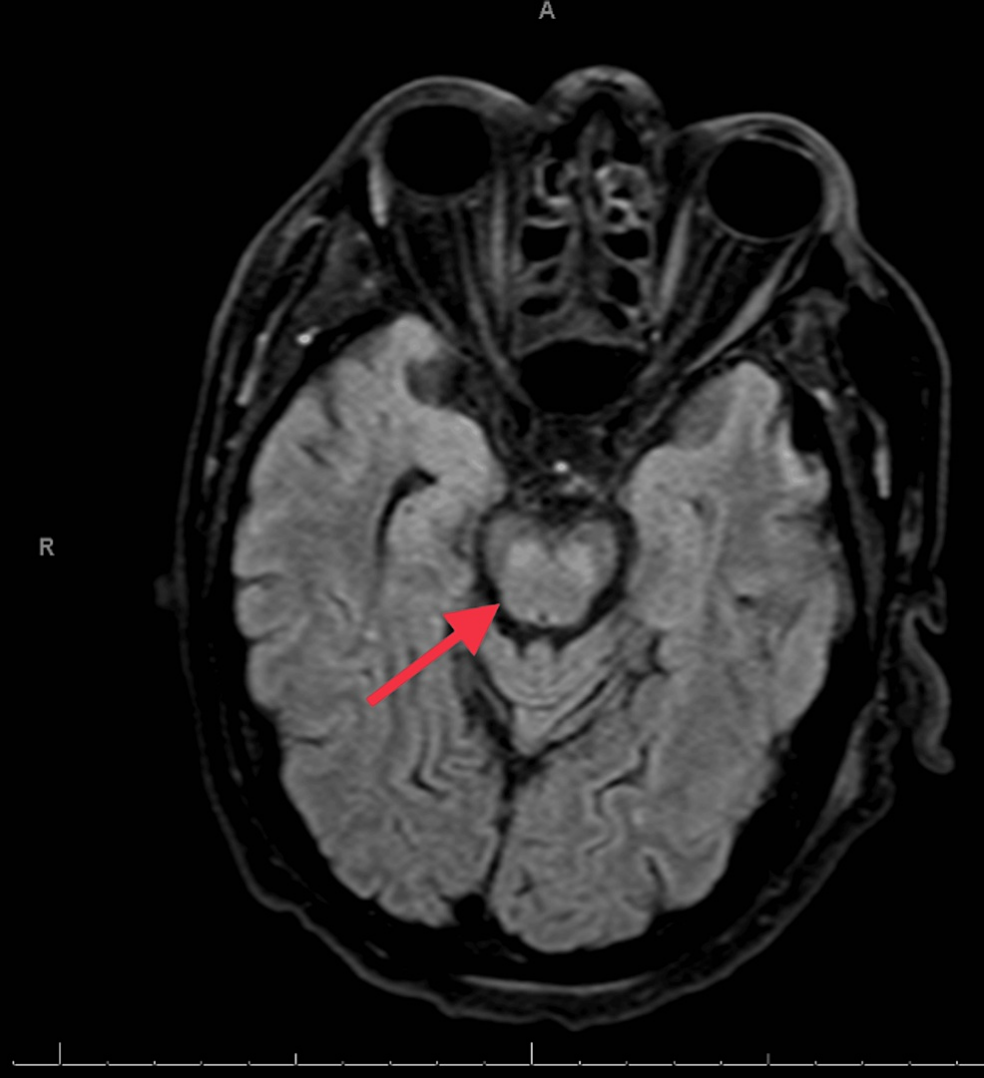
MRI T1 view coronal section showing acute central pontine myelinolysis
